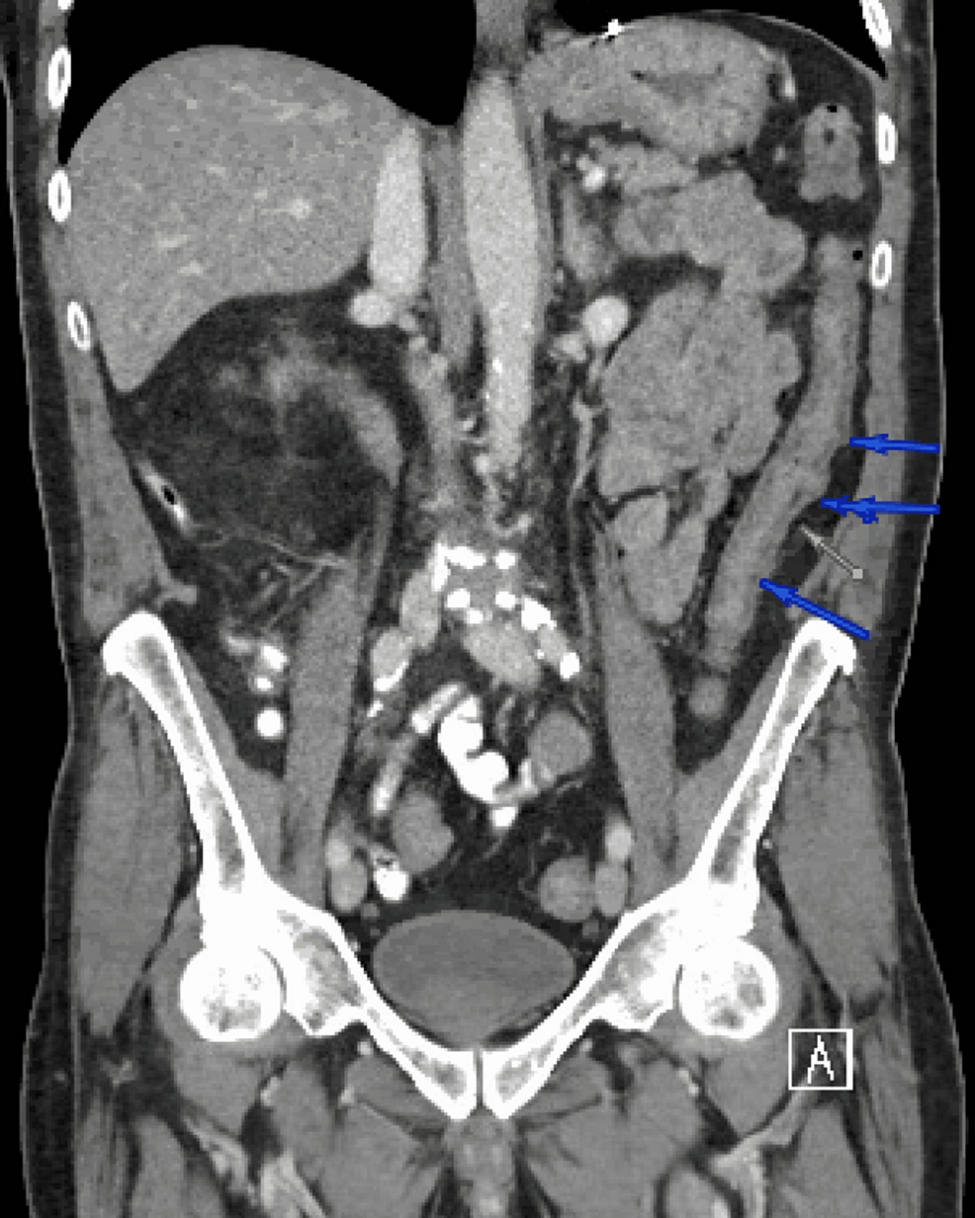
Computed tomography (CT) of abdomen and pelvis showing bowel wall thickening of descending colon
